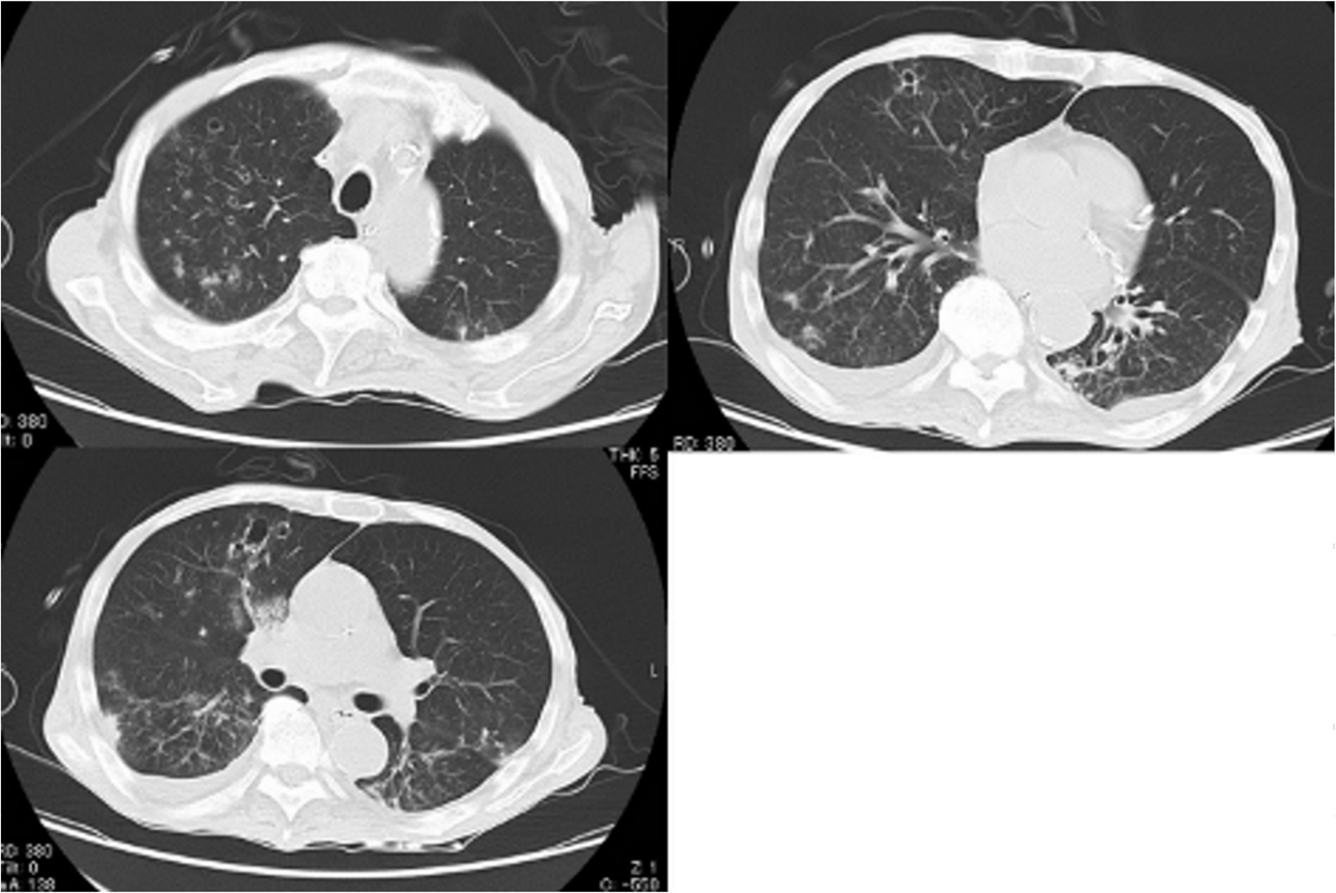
Chest CT after antifungal therapy. Chest CT images obtained on the 29^th^ day of admission showed that multiple small cavitary lesions, nodules and ground-glass opacity had improved after antifungal therapy.
